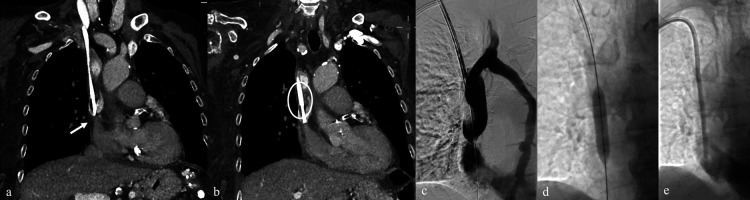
(a,b) Pre-removal CT of a TCC showing stenosis at the junction of the SVC and AD (arrow), associated with circumferential thrombotic deposition (circle). (c) Diagnostic venography performed via a 7 Fr introducer after TCC removal confirming CT findings, with collateral drainage through the hemiazygos vein. (d,e) Balloon angioplasty of the stenotic tract followed by successful placement of a new TCC.
